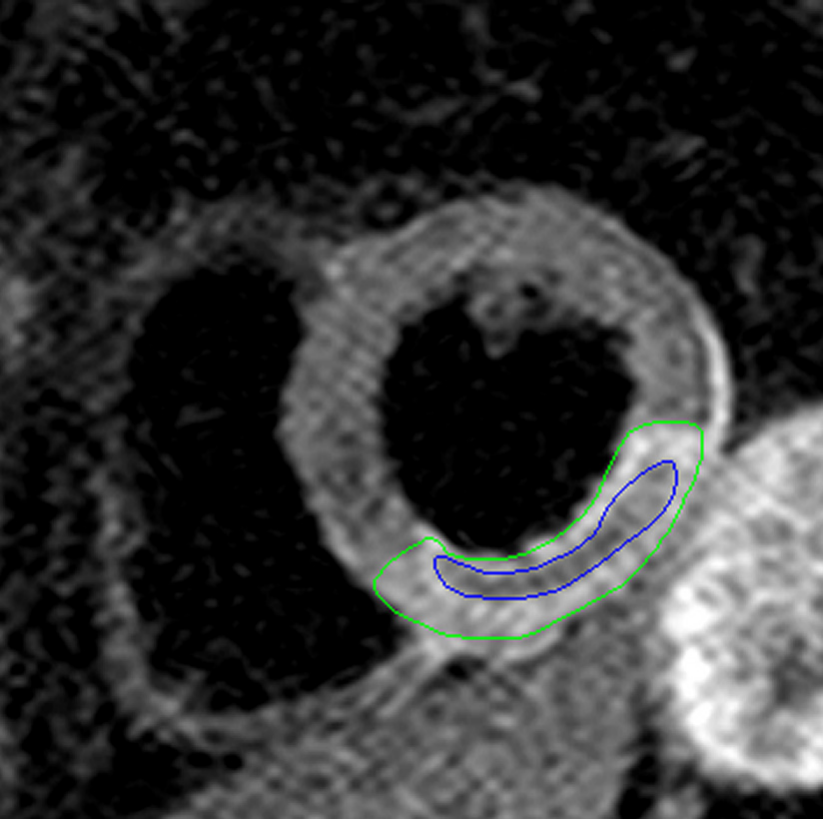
T2-weighted spin echo imaging in a patient with inferior/inferolateral STEMI due to occluded right coronary artery showing myocardial edema (green contours). The hypointense zones within the edematous region likely represent intramyocardial hemorrhage (blue contours).
